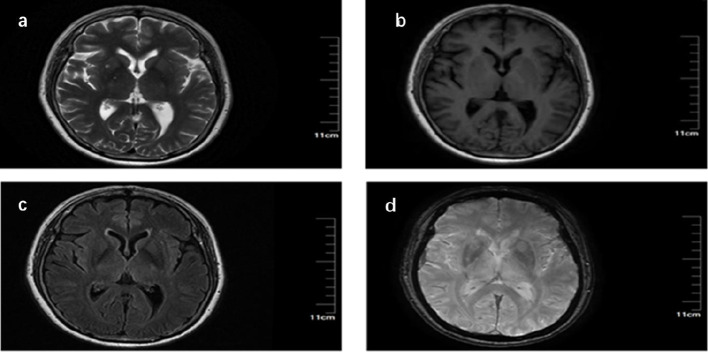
Magnetic Resonance Imaging (MRI) and Susceptibility-Weighted Imaging (SWI). (a) T2-weighted imaging (T2WI) shows small patchy hyperintensities in the bilateral frontal and parietal lobes, and a small patchy long T2 signal in the right basal ganglia region; (b) T1-weighted imaging (T1WI) reveals a long T1 signal in the right basal ganglia region; (c) Fluid-attenuated inversion recovery (FLAIR) imaging demonstrates a signal with hypointensity in the center and hyperintensity in the periphery in the right basal ganglia region, as well as rim-like hyperintensities around the bilateral lateral ventricles; (d) Susceptibility-weighted imaging (SWI) shows no abnormal hypointensities.
